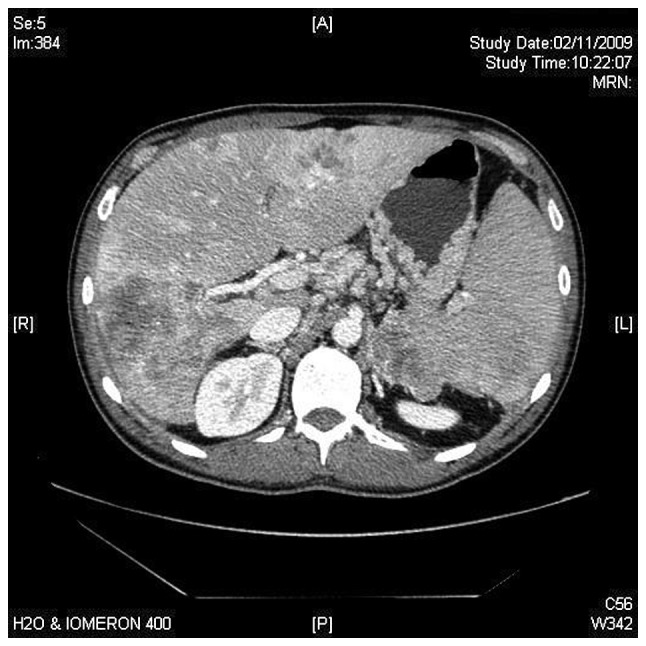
Abdominal CT images of patient 1 at diagnosis.
